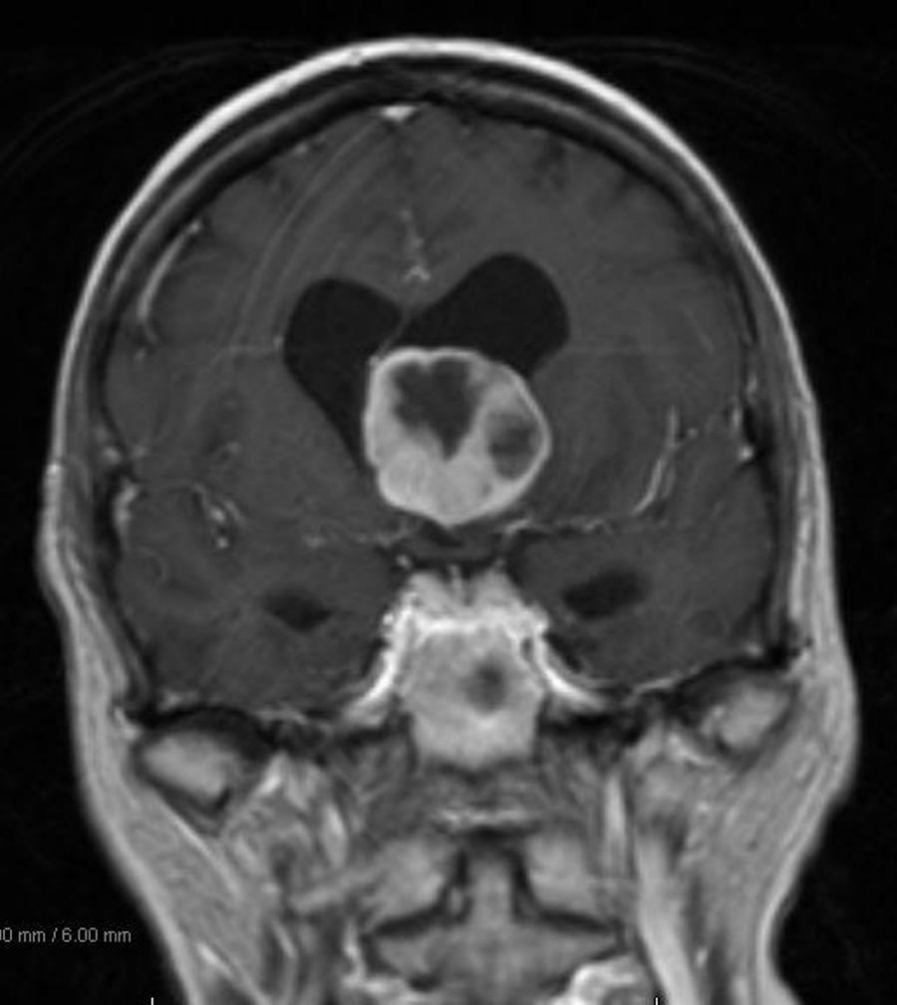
Initial MRI (post-contrast) Coronal post-contrast MRI demonstrating the mass effect caused by the heterogeneously enhancing lesion.
